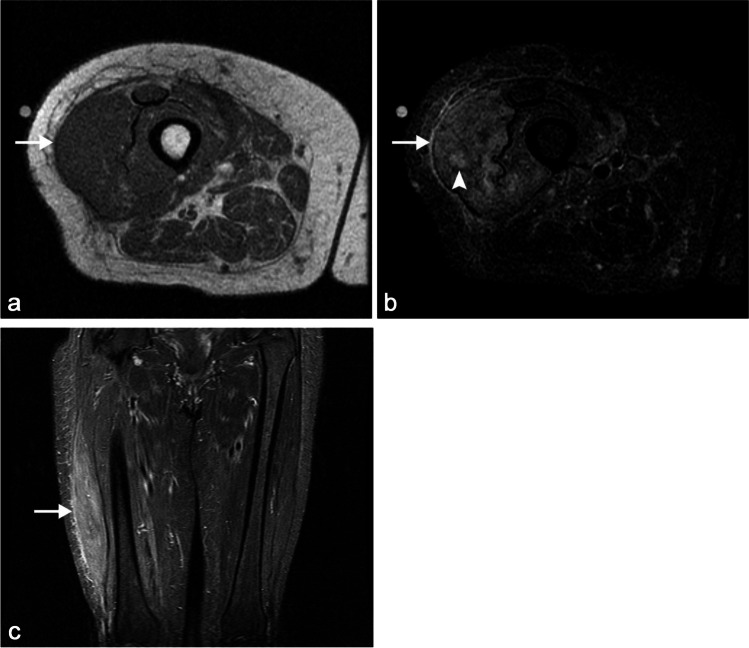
Fifty-five-year-old woman with poorly controlled type 2 diabetes mellitus with right thigh pain. Axial T1 (a), STIR (b), and coronal STIR (c) MR images demonstrate diffuse swelling and edema of the right vastus lateralis muscle (*arrows*) with a small focus of myonecrosis (*arrowhead*, b). The patient had no fever or leukocytosis and responded well to conservative management, differentiating this patient’s diagnosis of diabetic muscle infarct from pyomyositis, which can otherwise look similar on imaging
